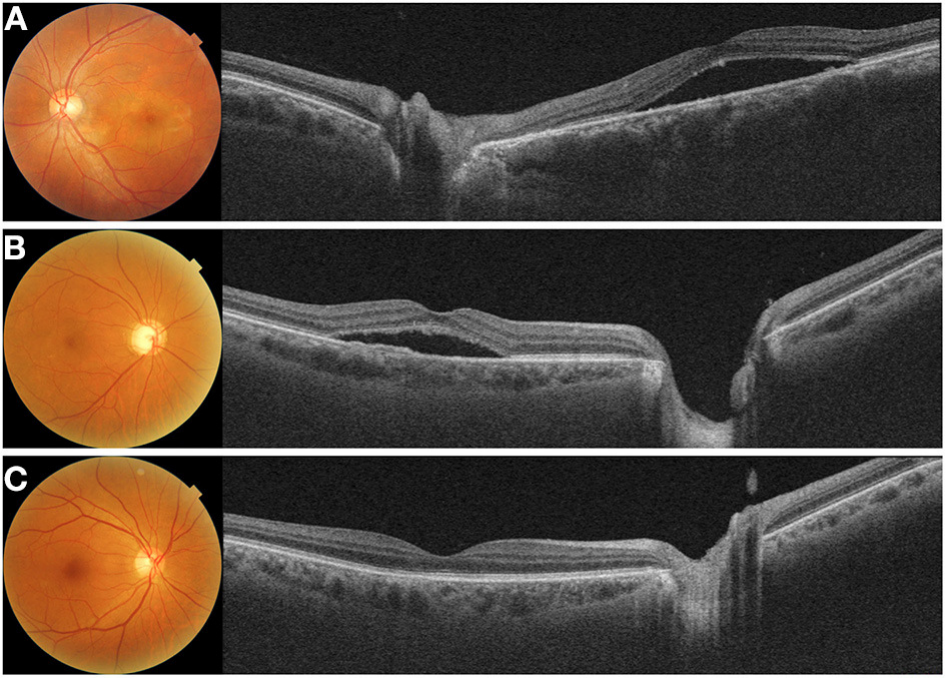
Representative cases with (A) acute central chorioretinopathy (CSC), (B) chronic CSC, and (C) resolved CSC. (A) A 38-year-old male with acute CSC showed diffuse thickening of both the macular and peripapillary choroid with dilated large choroidal vessels on a cross-sectional swept-source optical coherence tomography (SS-OCT) image. (B) A 69-year-old male with chronic CSC had increased subfoveal choroidal thickness (CT) and showed shallow irregular pigment epithelial detachment on and SS-OCT image. Peripapillary choroid was also thickened with dilated pachyvessels. (C) A 69-year-old male with resolved CSC showed diffuse thickening of the macular choroid. But, this patient had a relatively thinner peripapillary choroid.
